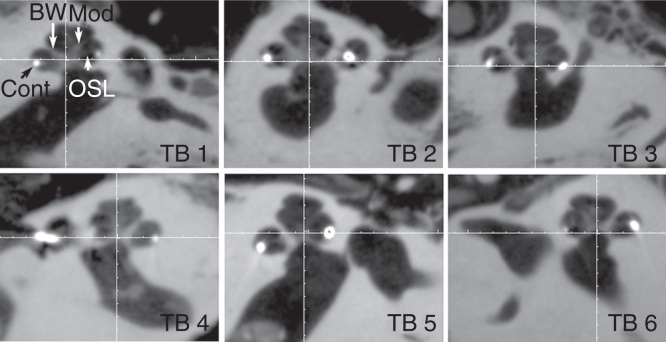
Fine cochlear structures demonstrated by cone-beam computed tomography (CBCT). All specified landmarks were clearly identified in all six temporal bones (TB). BW, bony wall; Cont, contours of cochlear implant; Mod, modiolus; OSL, osseous spiral lamina.
